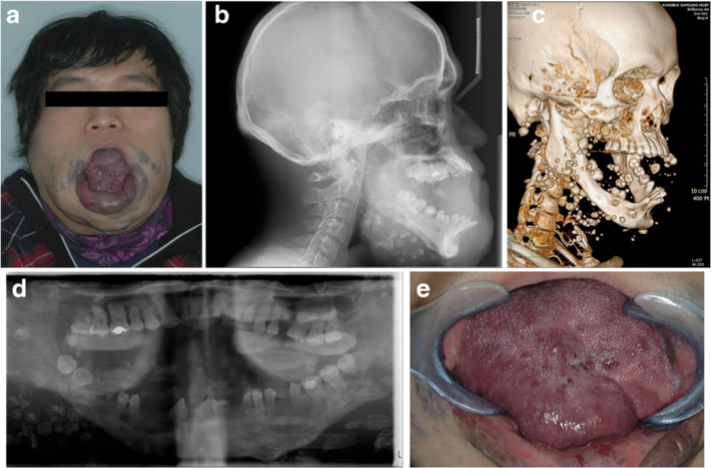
Preoperative patient information. a The tongue is interpositioned between the teeth, interfering with chewing. b The patient shows severe mandible prognathism, with anterior open-bite. c 3-D facial computed tomography view reveals the calcification to be away from the mandible body. d Panoramic image shows phleboliths around the mandible body. e Clinical photograph shows the macroglossia
